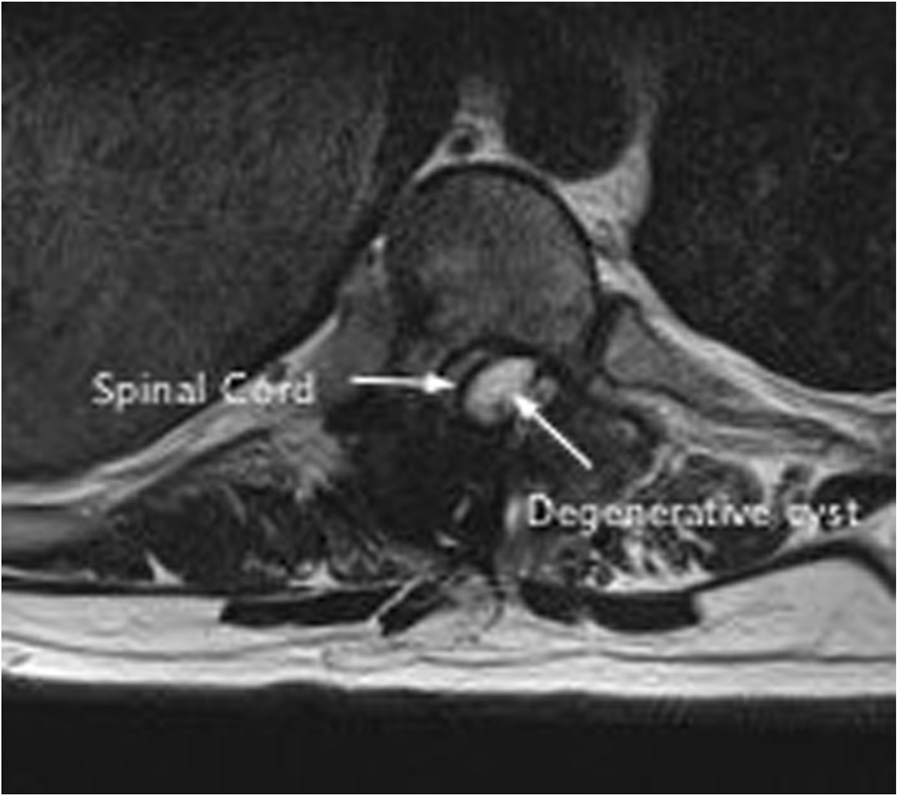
An axial magnetic resonance image of the cyst within the spinal canal and compression of the spinal cord.
